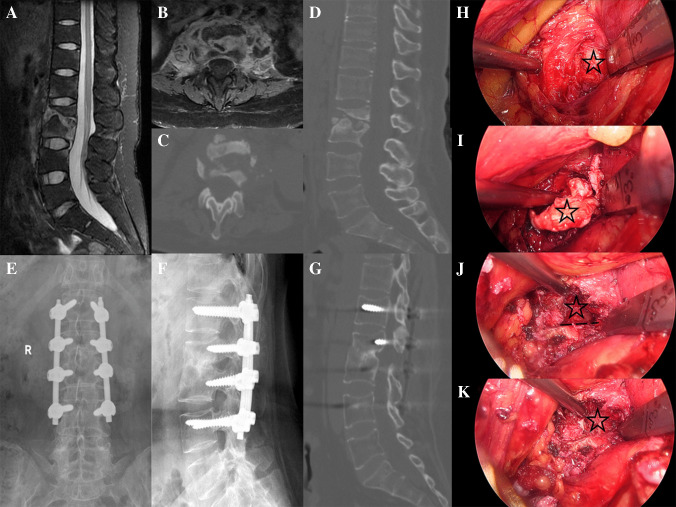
Case 6 A/B: PreoperativeMRI revealed abscess formation in the L2/3, with abscess formation both the psoas muscle. C/D: Preoperative CT demonstrated severe bone destruction in L2/3, with sequestrum formation in the L3 vertebra. E/F: X-ray at 1 month postoperatively. G:CT at 12 months postoperatively confirmed complete fusion at L2/3. H: Exposure of the lesion, with the lesion marked by a black pentagram. I: Clearance of the lesion. J: Preparation of the bone graft bed, with the bone surface indicated by a black dashed line. K: Implantation of allogeneic bone
